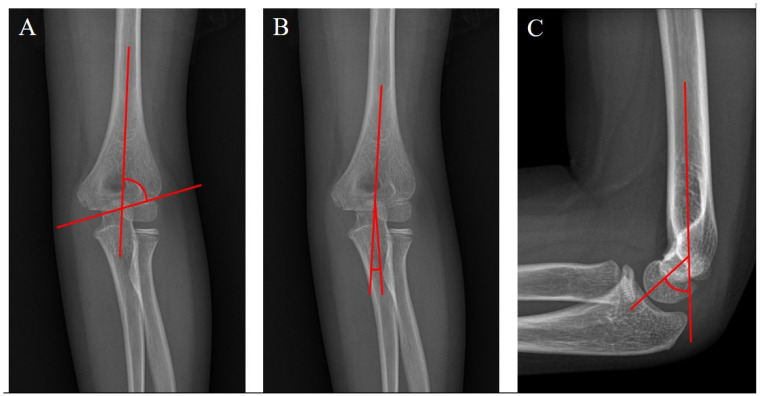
Angles for the evaluation of radiologic outcomes. (A) The Baumann angle was measured using the line parallel to the lateral condylar physis and the line perpendicular to the axis of the humeral shaft on AP radiographs. (B) The ulnohumeral angle was assessed using the ulnar and humeral length axes on AP radiographs. (C) The humerocapitellar angle was measured using the humeral length axis and the line parallel to the capitellum on lateral radiographs.
